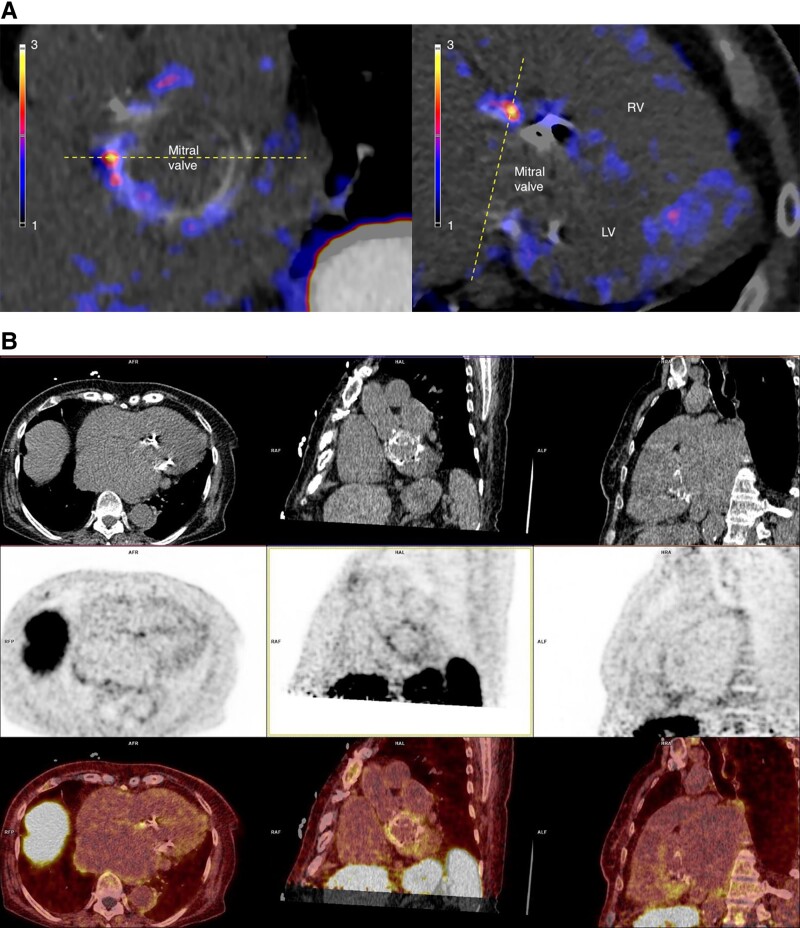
(*A*) [^64^Cu]Cu-DOTATATE PET/CT of Patient 1. Perpendicular positron emission tomography/computed tomography fusion images of the mitral valve revealing a focal high-tracer uptake of [^64^Cu]Cu-DOTATATE on the anterior aspect of the mitral valve prosthesis. The myocardium in the ventricle has very low tracer uptake. The dotted lines indicate two image planes. LV, left ventricle; RV, right ventricle. (*B*) Positron emission tomography/computed tomography images from Patient 1 showing an uptake of [^64^Cu]Cu-DOTATATE in cross-sectional, coronal, and sagittal views, respectively. Top row: computed tomographyCT, middle row: positron emission tomography images; bottom row: fused positron emission tomography /computed tomography images.
